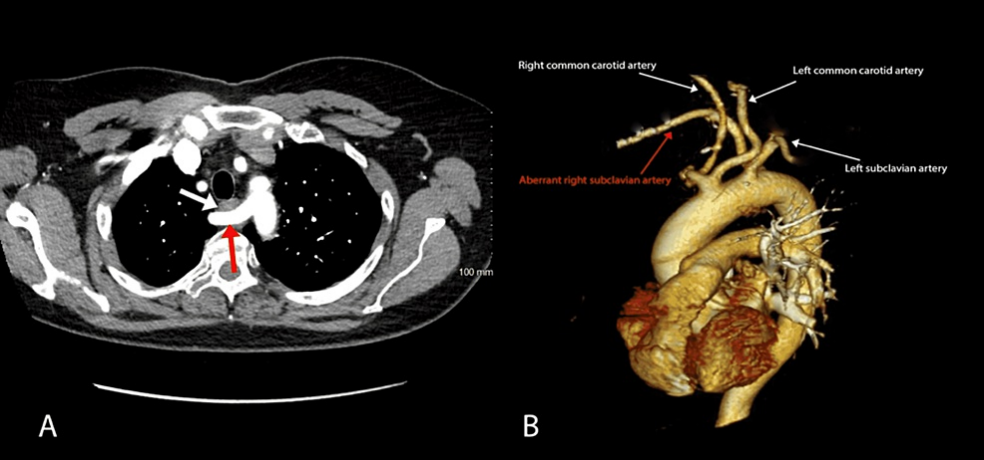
(A) CT angiography scan showing aberrant right subclavian artery (red arrow) coursing posterior to the esophagus (white arrow), resulting in extrinsic compression. (B) Three-dimensional CT scan showing the heart and aortic arch. The right subclavian artery (highlighted in red) arises as the last branch from the aortic arch distal to the origin of the left subclavian artery.
